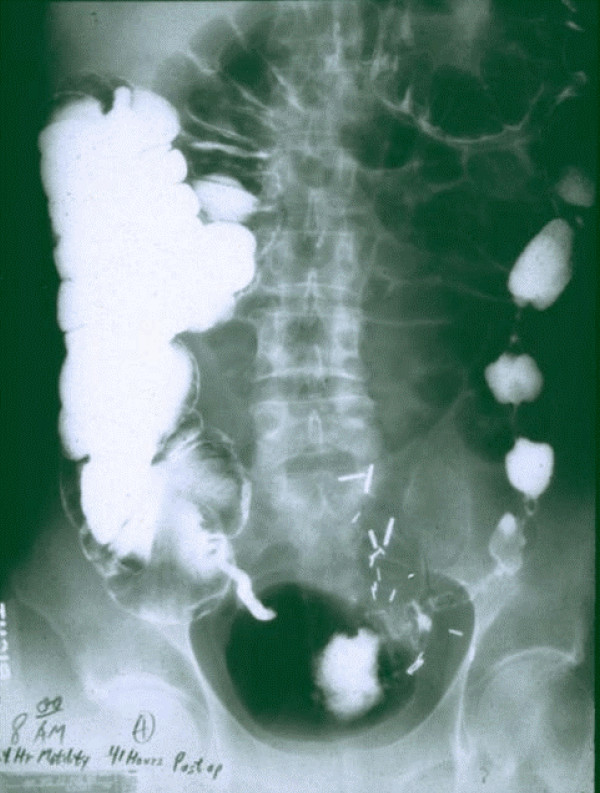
BaSO4 has traversed the secure and patent stapled anastomosis, to outline the rectum. The patient ate his Thanksgiving meal, and was discharged after a bowel movement within 48 hours of surgery. The X-ray shows a normal peristaltic pattern despite surgical trauma to the tissue. 8:00 am – 24 hour motility, 41 hours after sigmoid resection.
